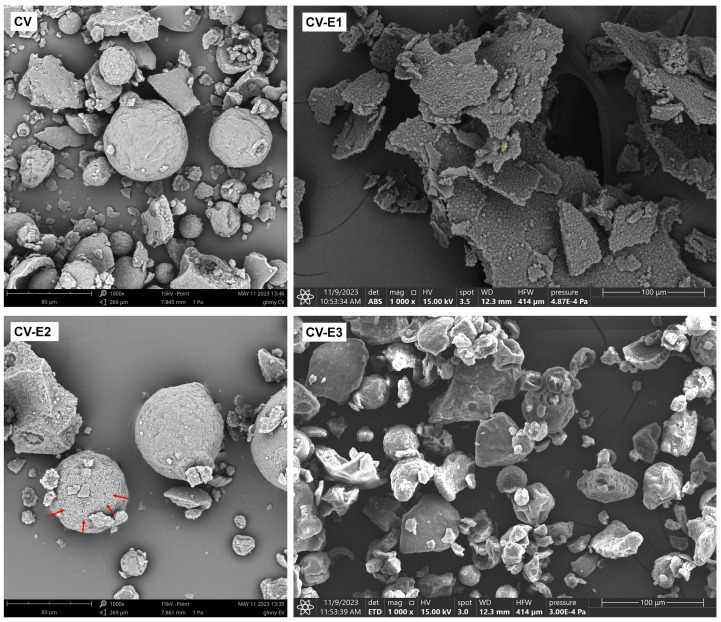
SEM images of dry *Chlorella* powder (CV), *Chlorella* powder after sonication (CV-E1), *Chlorella* powder after Soxhlet extraction (CV-E2), and *Chlorella* powder after ultrasound-assisted solvent extraction (CV-E3), magnification 1000×. Red arrows indicate pores formed on the surface of cellular aggregates following Soxhlet extraction. The yellow “+” symbol visible in the center of the CV-E1 image is a software-generated marker and has no physical or analytical significance.
